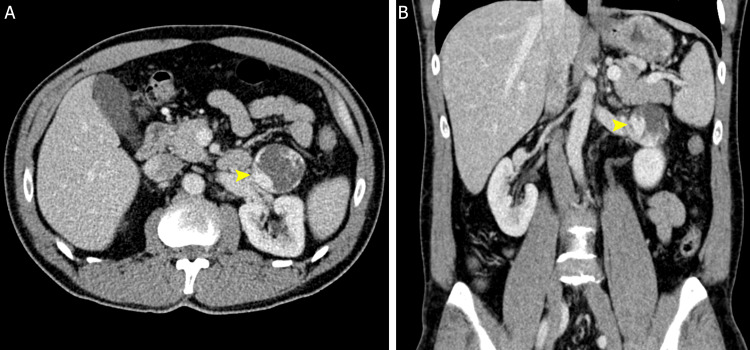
Abdominal CECT portal venous phase, axial plane (A), and coronal reconstruction (B) Located in the left perirenal space and anterior to the renal vessels, a round circumscribed mass (yellow arrows) measuring 4.5 cm x 4 cm (transverse x anteroposterior) with discontinuous peripheral nodular enhancement and a center of lower density for the acquisition phase. No phleboliths were observed in the mass, and there were no signs of thrombosis, vascular invasion, adenomegaly, or metastatic lesions. CECT: Contrast-enhanced computed tomography
